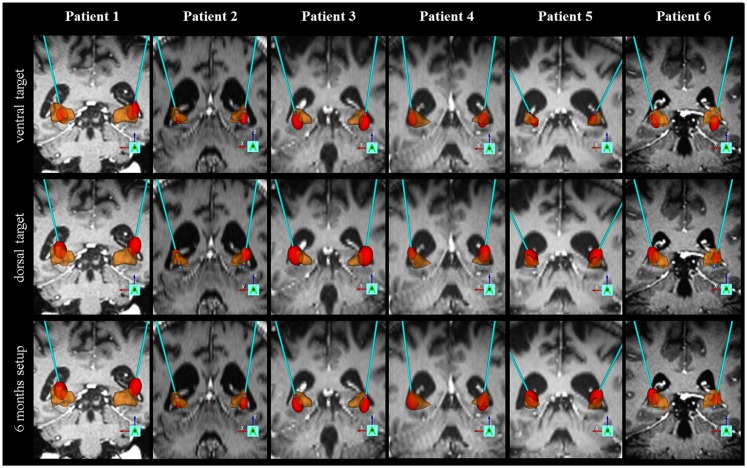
Individual electrode localization. Visualization on 3D coronary MRI-view of individual electrodes and volume of tissue activated (VTA, in red) in relation to the pallidum (in brown) for the ventral target (GPI), the dorsal target (GPE) and the target at 6-month follow-up.
